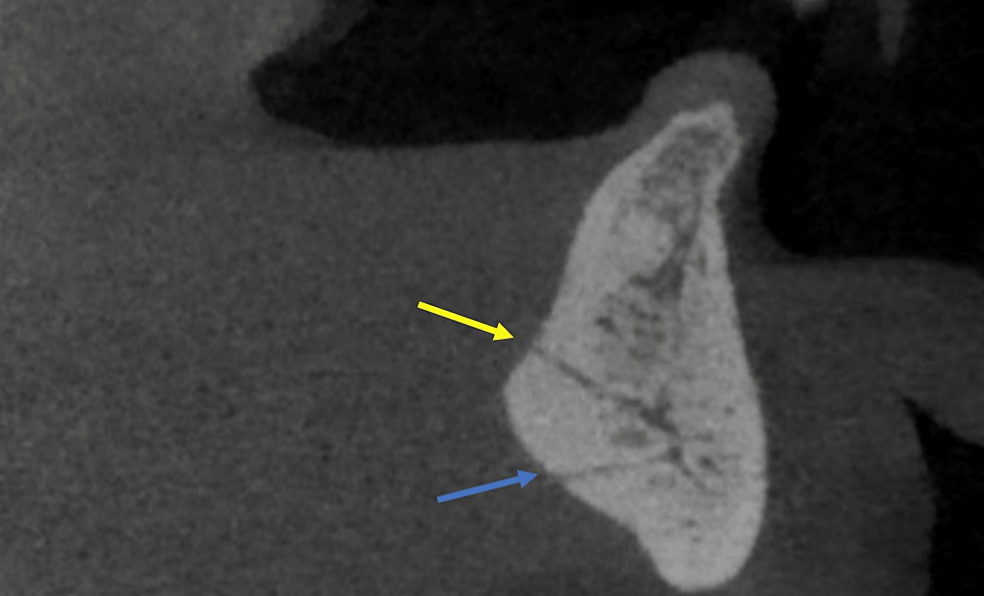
Sagittal cross-section of the CBCT scan showing superior and inferior levels of the lingual artery entering and exiting the lingual cortical plate. CBCT, cone beam computed tomography
